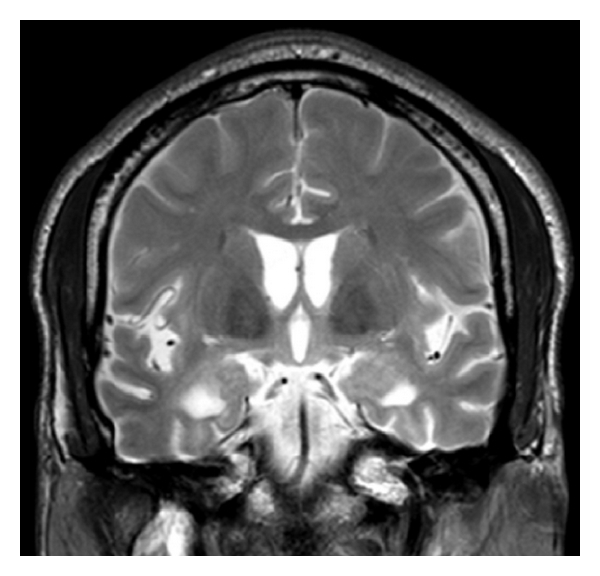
T2-weighted image on the coronal plane showing cortico-subcortical bilateral hyperintensity involving the mesiotemporal region, including amygdala and hippocampi with sulci and temporal horns enlargement.
